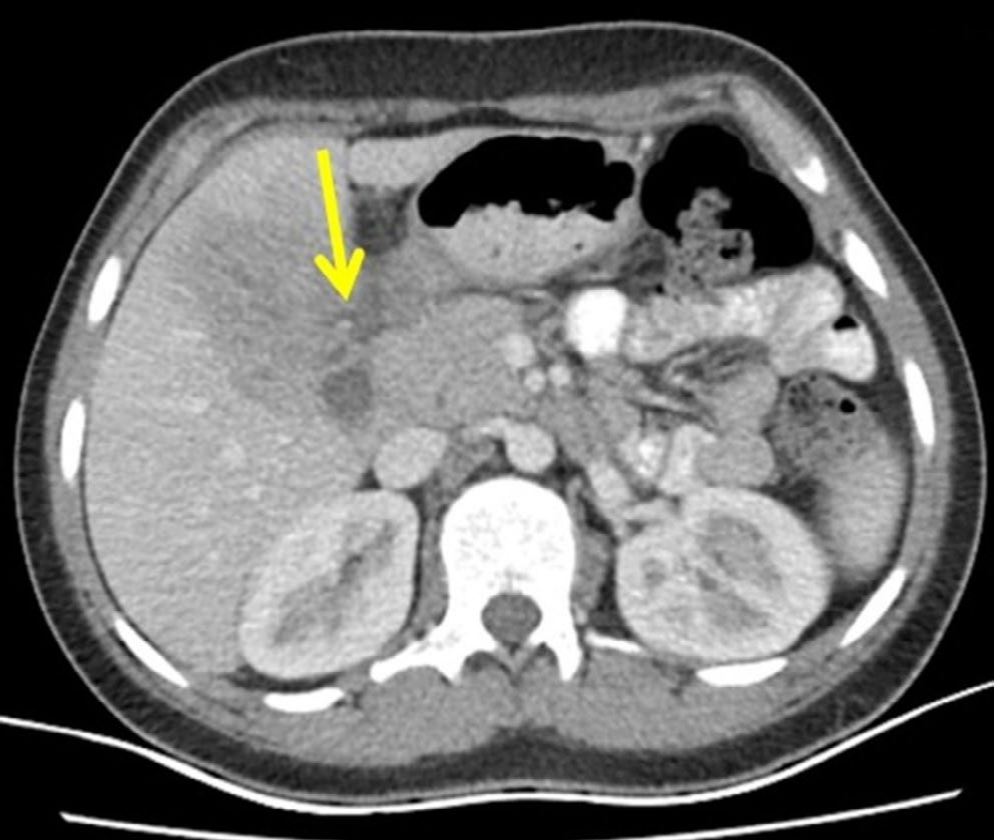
Axial CT demonstrates a multiloculated abscess in the left hepatic lobe (yellow arrow) abutting the gastric antrum, raising suspicion for fistulization.
